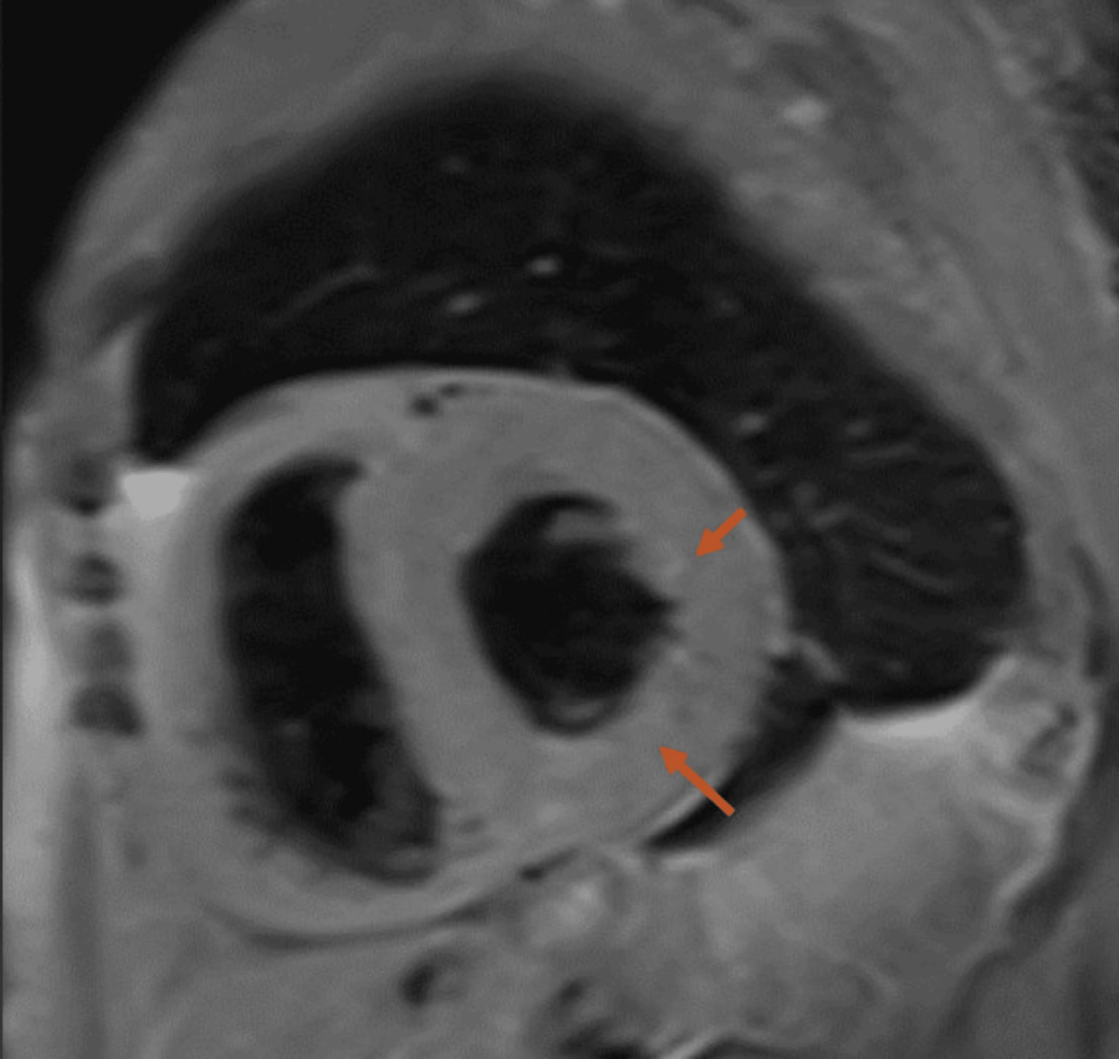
T2-weighted cardiac MRI image revealing concentric hypertrophy (arrows) without myocardial edema. The absence of myocardial edema on cardiac MRI, despite the presence of late gadolinium enhancement and left ventricular wall thickening, is a crucial finding that points toward HCQ-induced cardiomyopathy MRI: magnetic resonance imaging, HCQ: hydroxychloroquine
